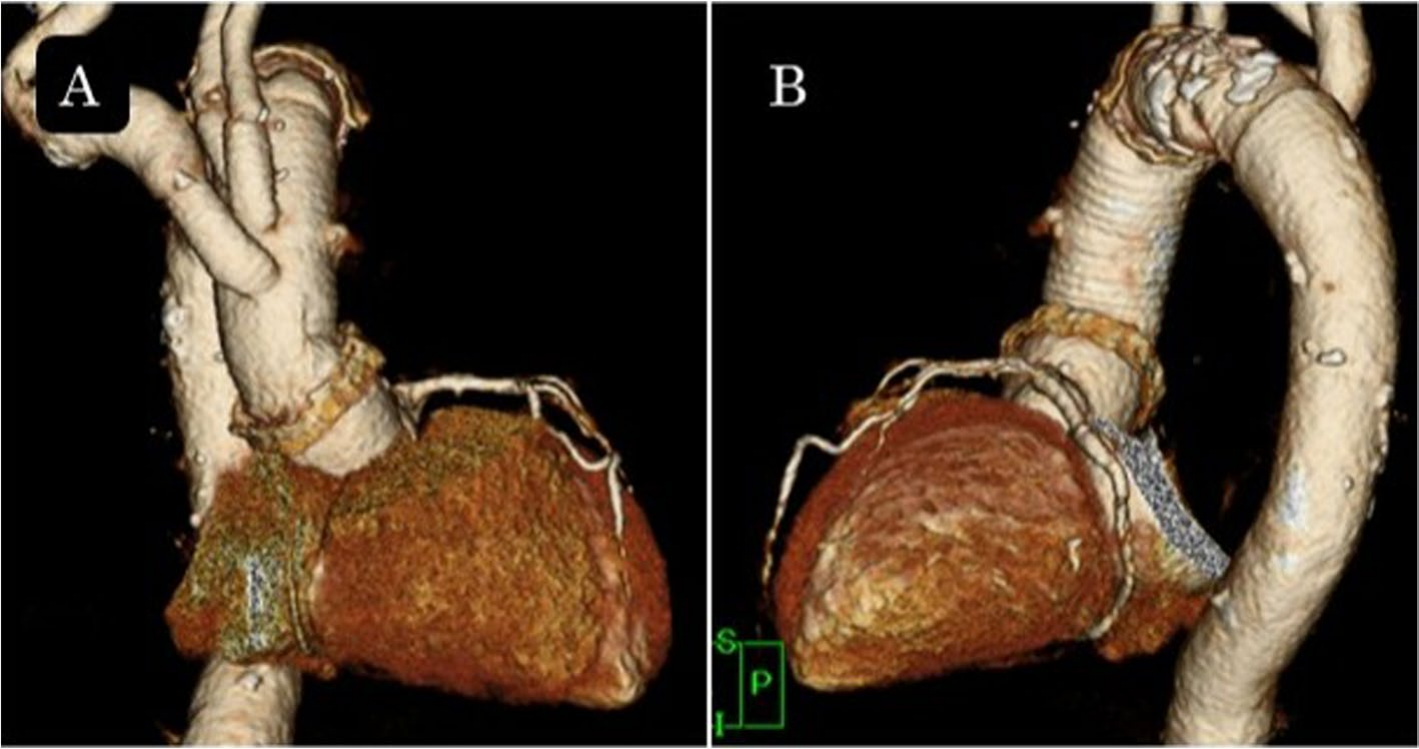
3D computed tomography image taken after the surgery: front view (A) and left lateral view (B)
